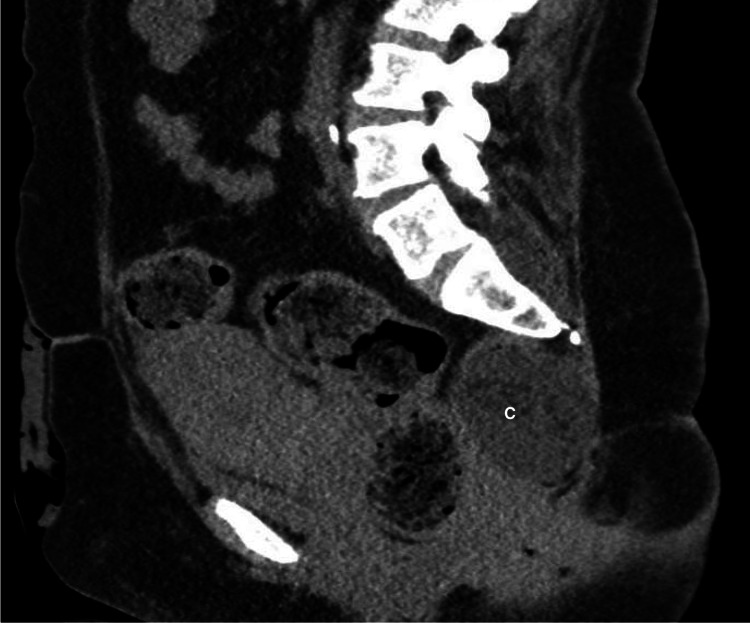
Noncontrast computed tomography intravenous pyelogram sagittal 2016. Teratoma measuring 54 mm × 49 mm × 46 mm (C).
